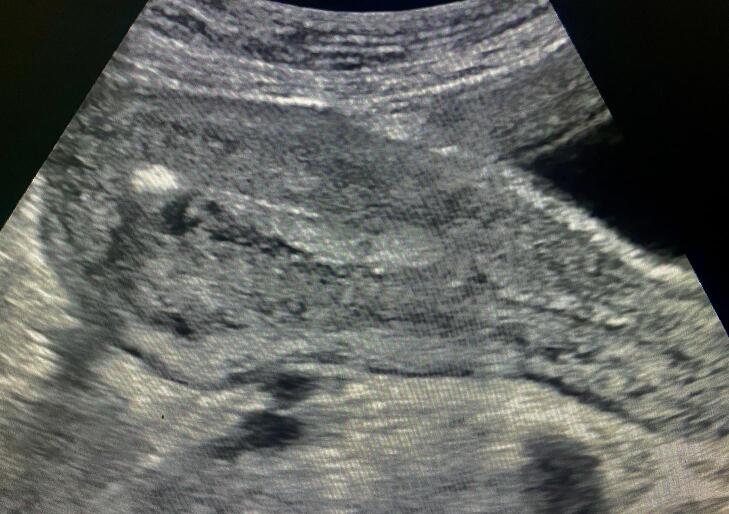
Sonographic image of maggots located high in the uterine cavity.
